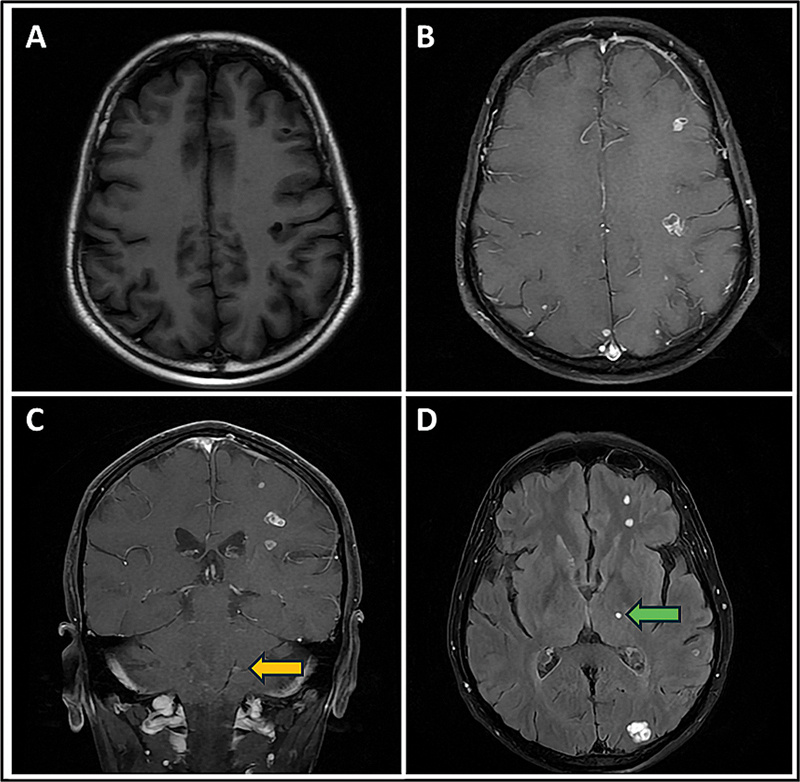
Axial T1-weighted image (
